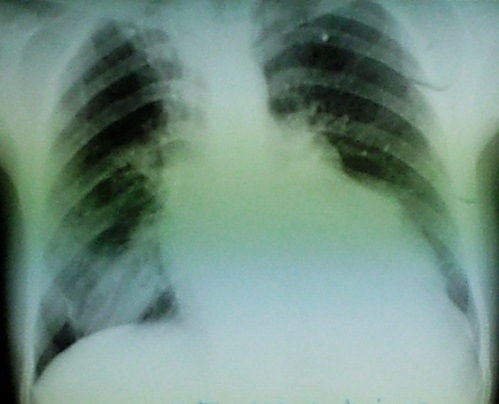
Radiographie thoracique montrant une cardiomégalie avec un bord gauche polylobé et une opacité basale droite
